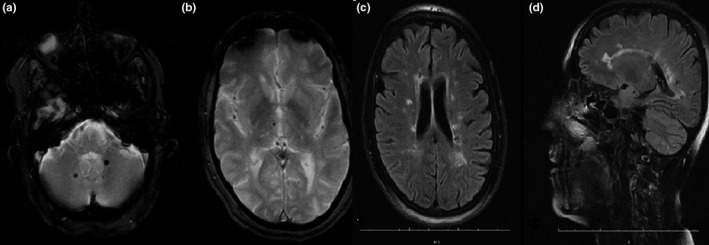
In case 7, gradient echo MRI showed signal loss consistent with multiple hemosiderin deposits (hypertensive microhemorrhage? amyloid angiopathy?) in both cerebellar hemispheres (a), thalamus (b), pons, right hemisphere, left lentiform nucleus, and left frontal gray–white matter. In axial (c) and sagittal (d) FLAIR sequences, hyperintense signal changes, some of which were located vertically to the callososeptal interface, were observed in bilateral periventricular deep white matter
